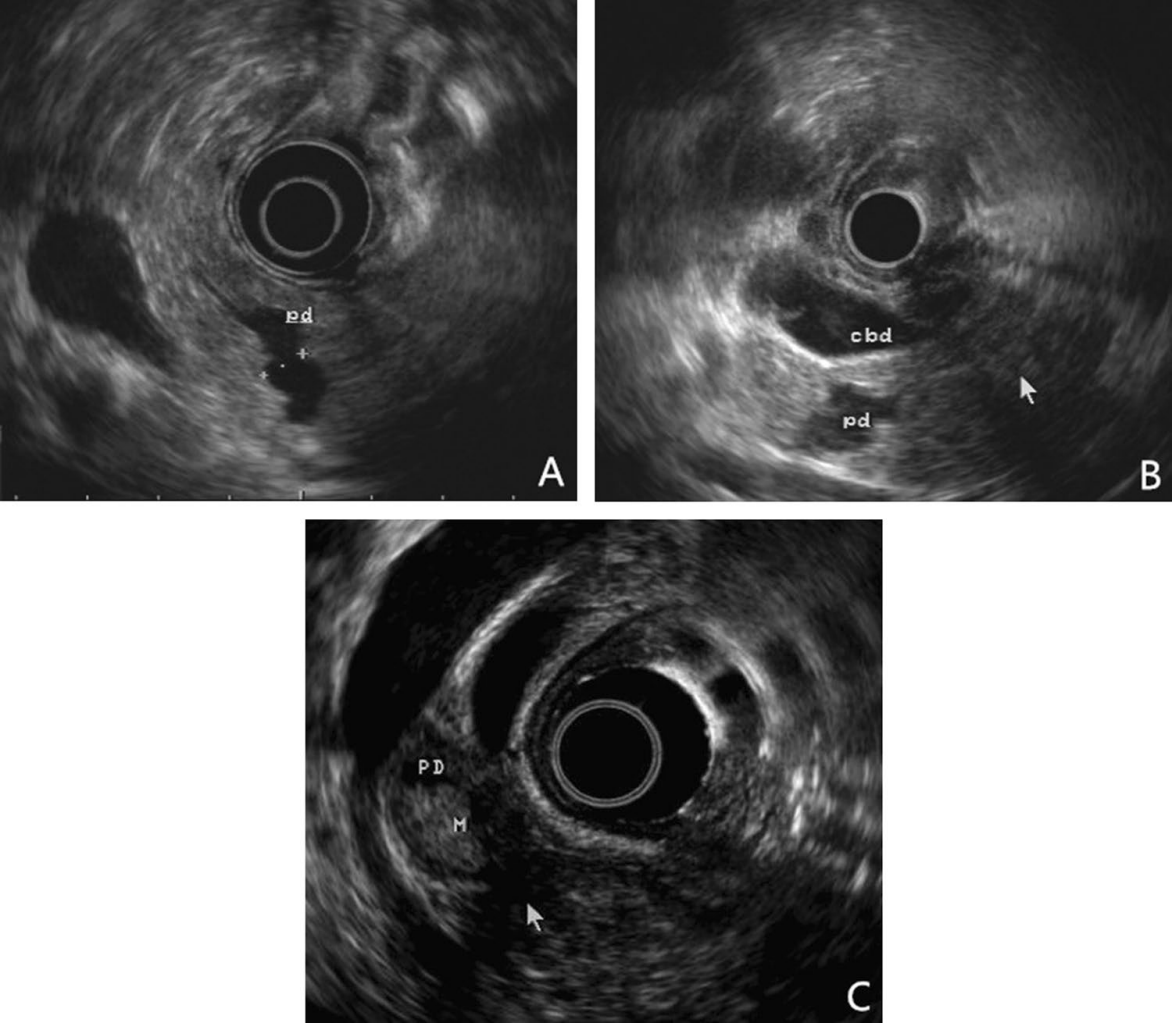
EUS images of IPMN with different pathology type. A Low-grade dysplasia. A cyst without mural nodules communicates with a non-dilated MPD. B High-grade dysplasia. A cystic communicates with dilated MPD without mural nodules. CBD is also dilated. C Invasive carcinoma. A mural nodule inside a dilated MPD. *EUS* endoscopic ultrasound, *IPMN* intraductal papillary mucinous neoplasm, *MPD* main pancreatic duct, *CBD* common bile duct
